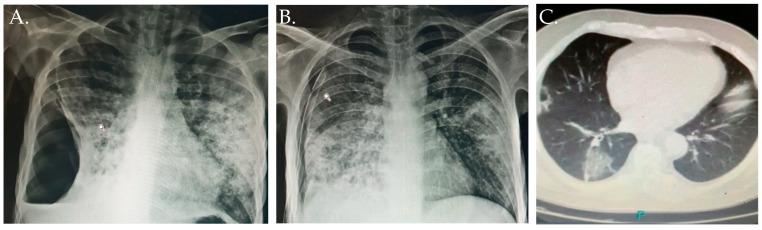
Chest radiography studies. (A,B) shows chest radiography study of Case 2. (A) Chest radiography of a 31-year-old male showed many small, thick-walled cavities bilaterally; evidence of perilesional ground glass haziness with air space opacities, right pneumothorax about 40%, pleural effusion, and many fibro-parenchymal opacities; a right chest drain tube was inserted (B). (C) shows a chest CT scan in a 67-year-old male (Case 3) revealing a right cavitary lesion, evidence of perilesional ground glass bilaterally with the largest lesion in the right lower lobe, and consolidative pulmonary opacities.
